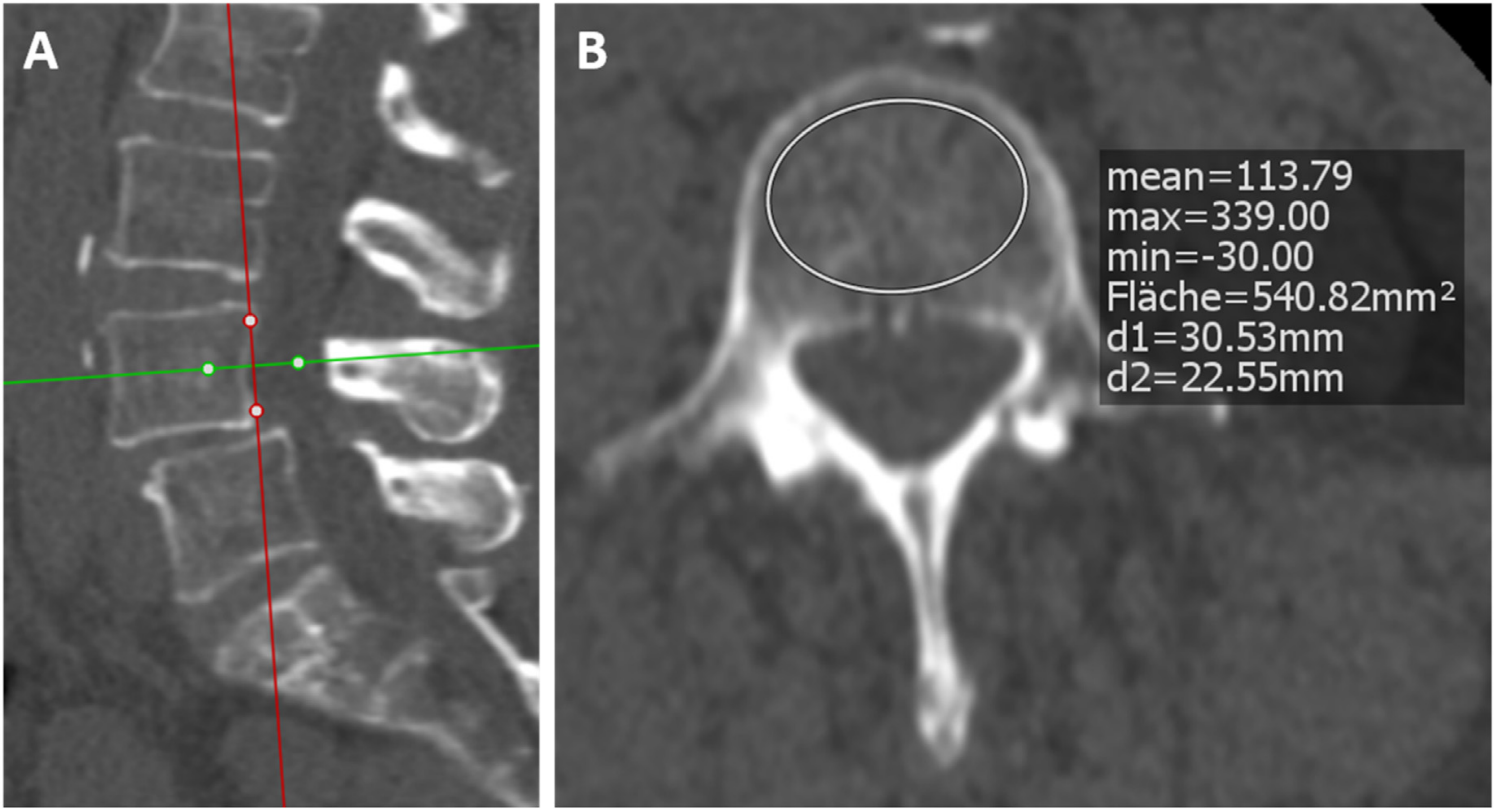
Demonstrating HU measurement in lumbar spine CTs. HU measurement in a lumbar spine CT scan in the A sagittal and B axial plane using an oval region of interest (ROI) over the axial image of the vertebral mid-body area. Phönix-PACS MERLIN Diagnostic Workcenter software automatically calculates the mean CT HU for the ROI in the axial plane.
